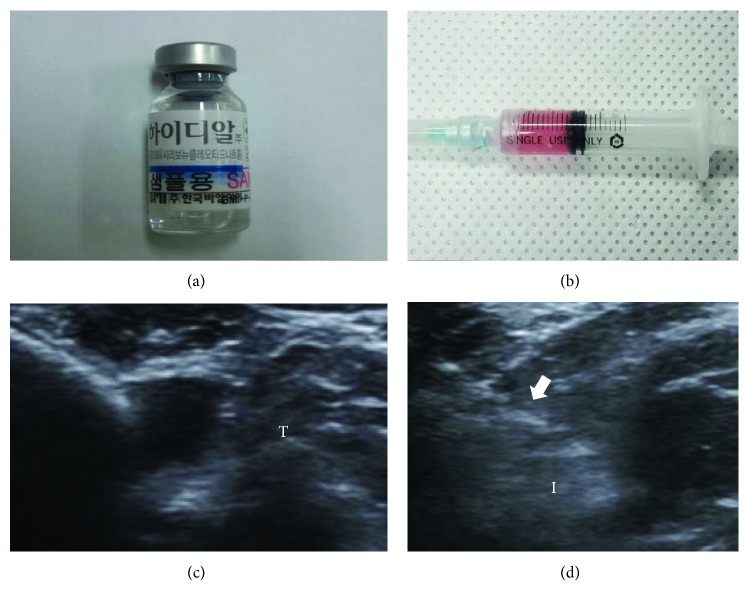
Human umbilical cord blood-derived mesenchymal stem cells (MSCs), polydeoxyribonucleotide (PDRN), and ultrasound images. (a) PDRN. (b) MSCs. (c) Injection was made in the left shoulder subscapularis full-thickness tears under ultrasound guidance. (d) Longitudinal ultrasound image shows the needle (arrow) in the left shoulder subscapularis of the rabbit. T: tendon; I: injectant.
